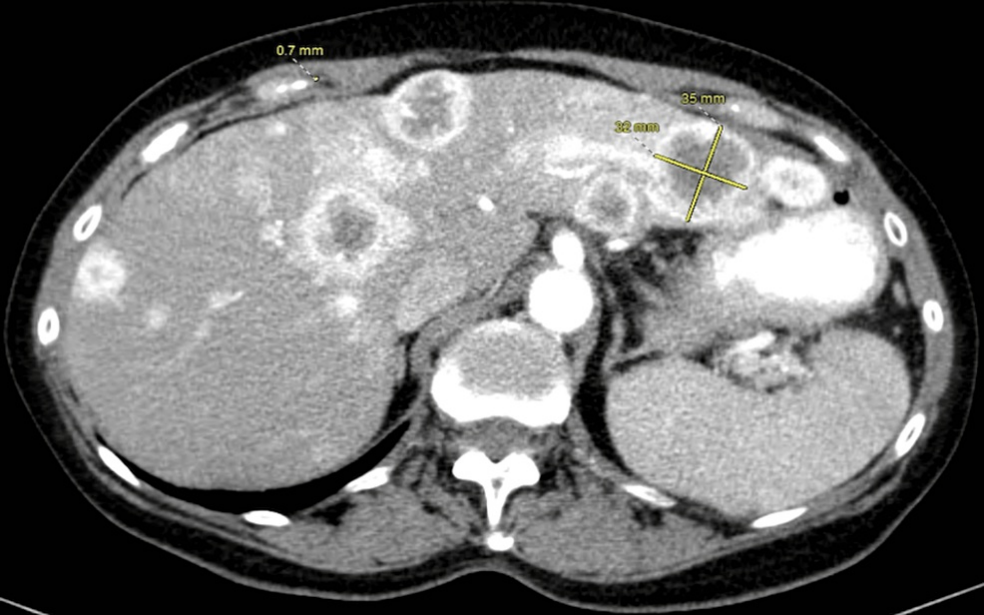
CT scan of the abdomen showing liver lesion in the lateral left hepatic lobe measuring 3.5 × 3.2 cm. CT: computed tomography
